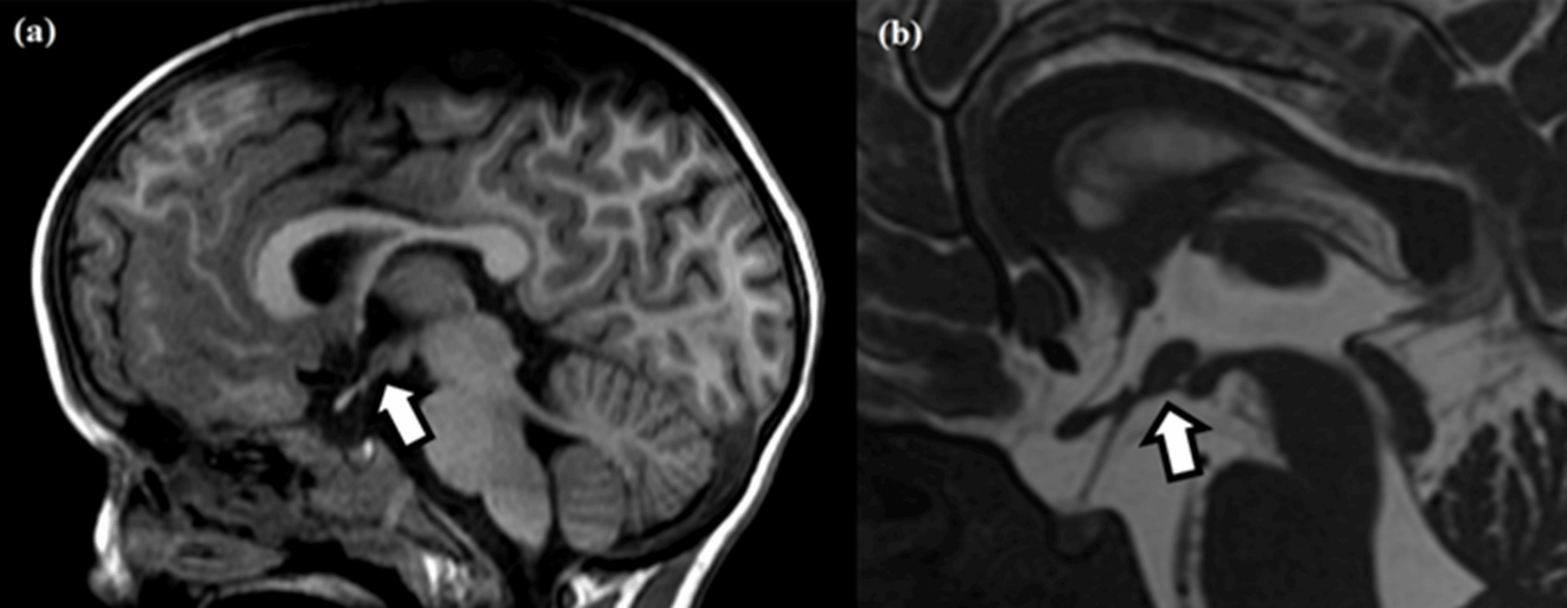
T1-weighted (a) and fast imaging employing steady-state acquisition (b) sagittal MRIs. Slight thinning of the corpus callosum isthmus and an oval formation with signal intensity similar to gray matter noted in the tuber cinereum, connecting both sides of the hypothalamus (white arrows).
